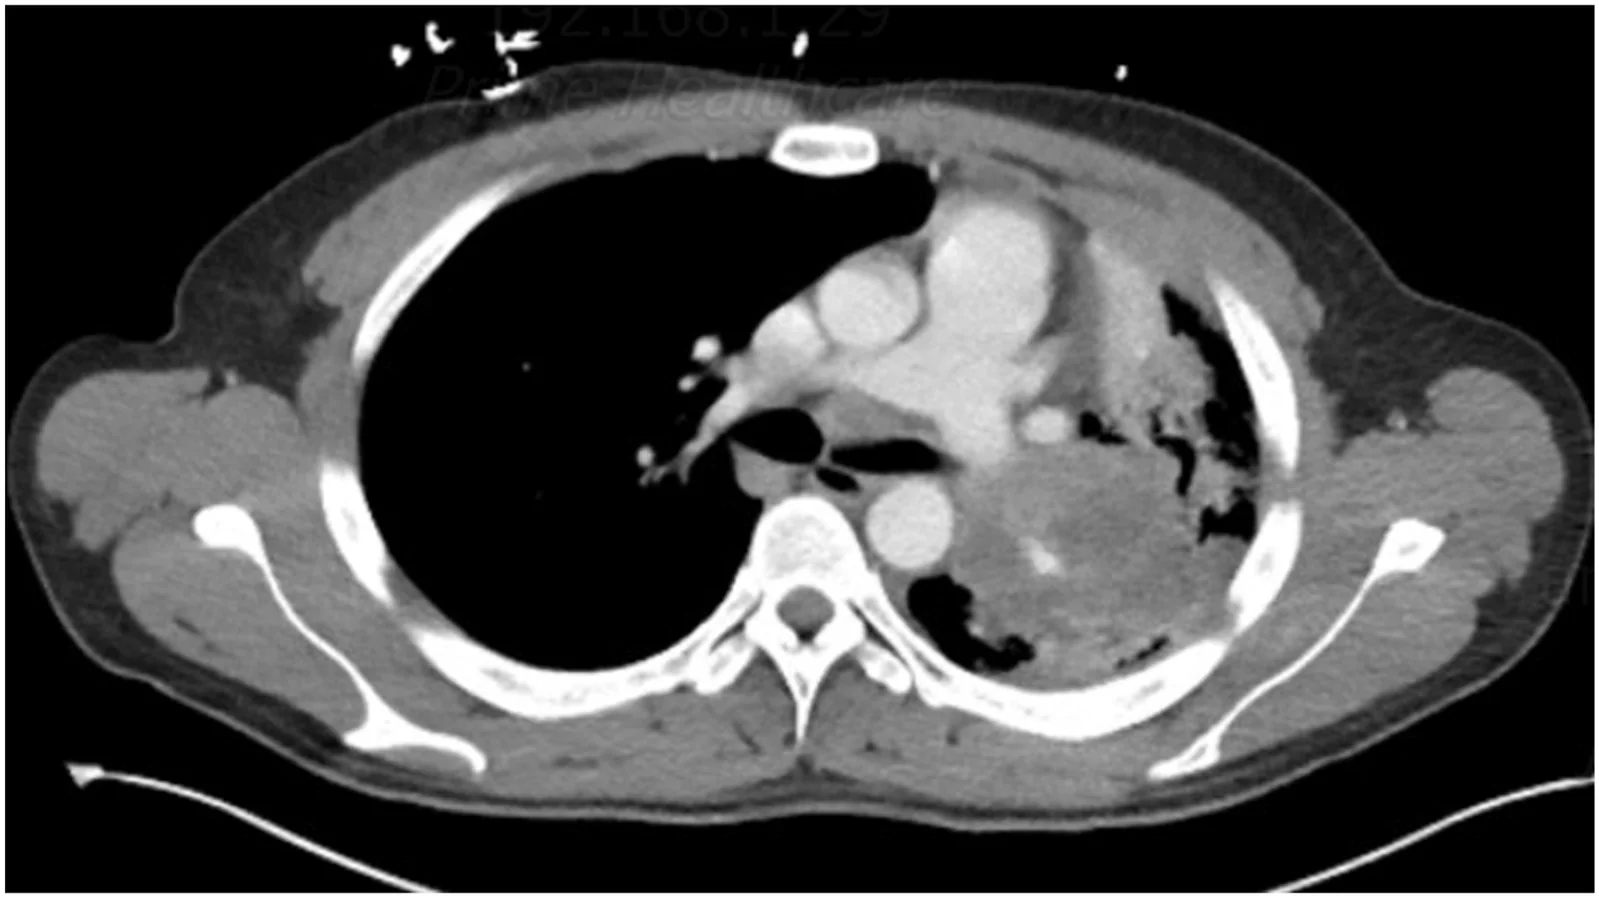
Contrast-enhanced computed tomography (CT) of the chest demonstrating a progressive left hilar mass measuring 6.8 × 5.5 cm
